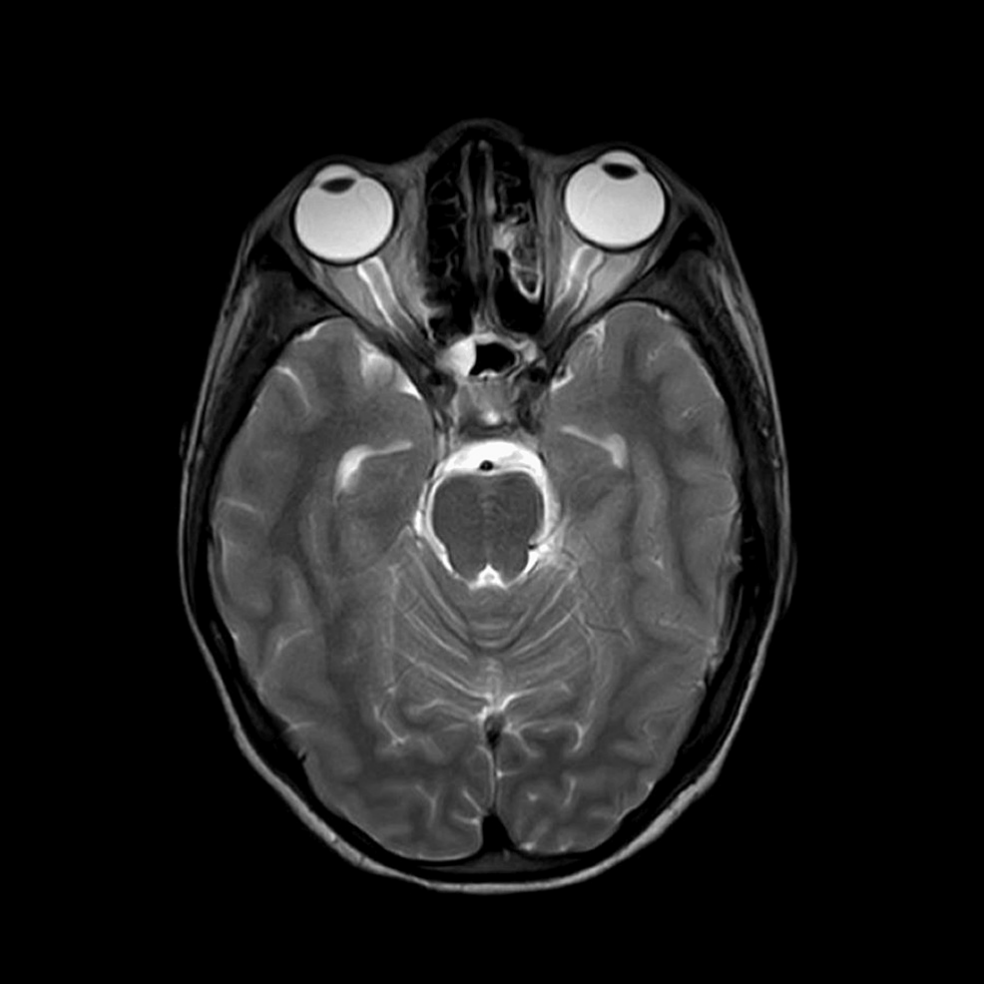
MRI The circle shows hyperintensity noted (right > left).
